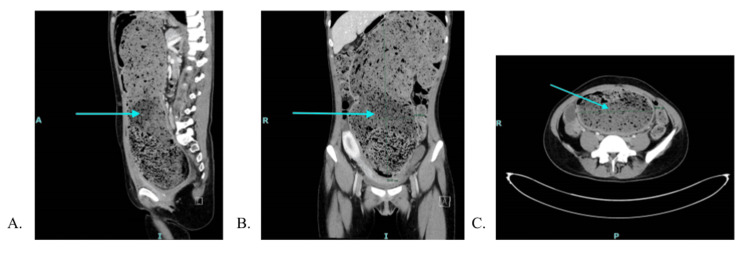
CT abdomen/pelvis with IV contrast on the day of admission, demonstrating a distended colon A: Sagittal view. B: Coronal view. C: Transverse view. The blue arrow points at the most distended portion of the colon.
